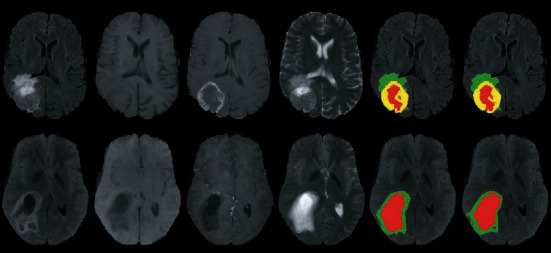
Example MRI from BraTS dataset.
